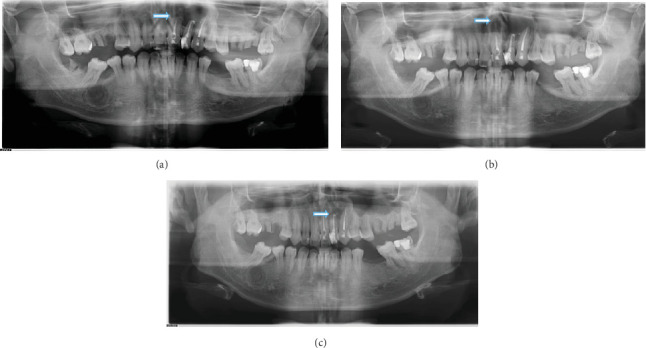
(a) Follow-up OPG X-ray 1 month after surgery showed a radiolucent area in the cyst defect decreased from the preoperative cyst size. (b) Follow-up OPG X-ray 3 months after surgery showed a radiolucent area in the cyst defect, smaller than the OPG X-ray 1 month after surgery. (c) Follow-up OPG X-ray 6 months after surgery showed a radiolucent area in the cyst defect getting smaller.
